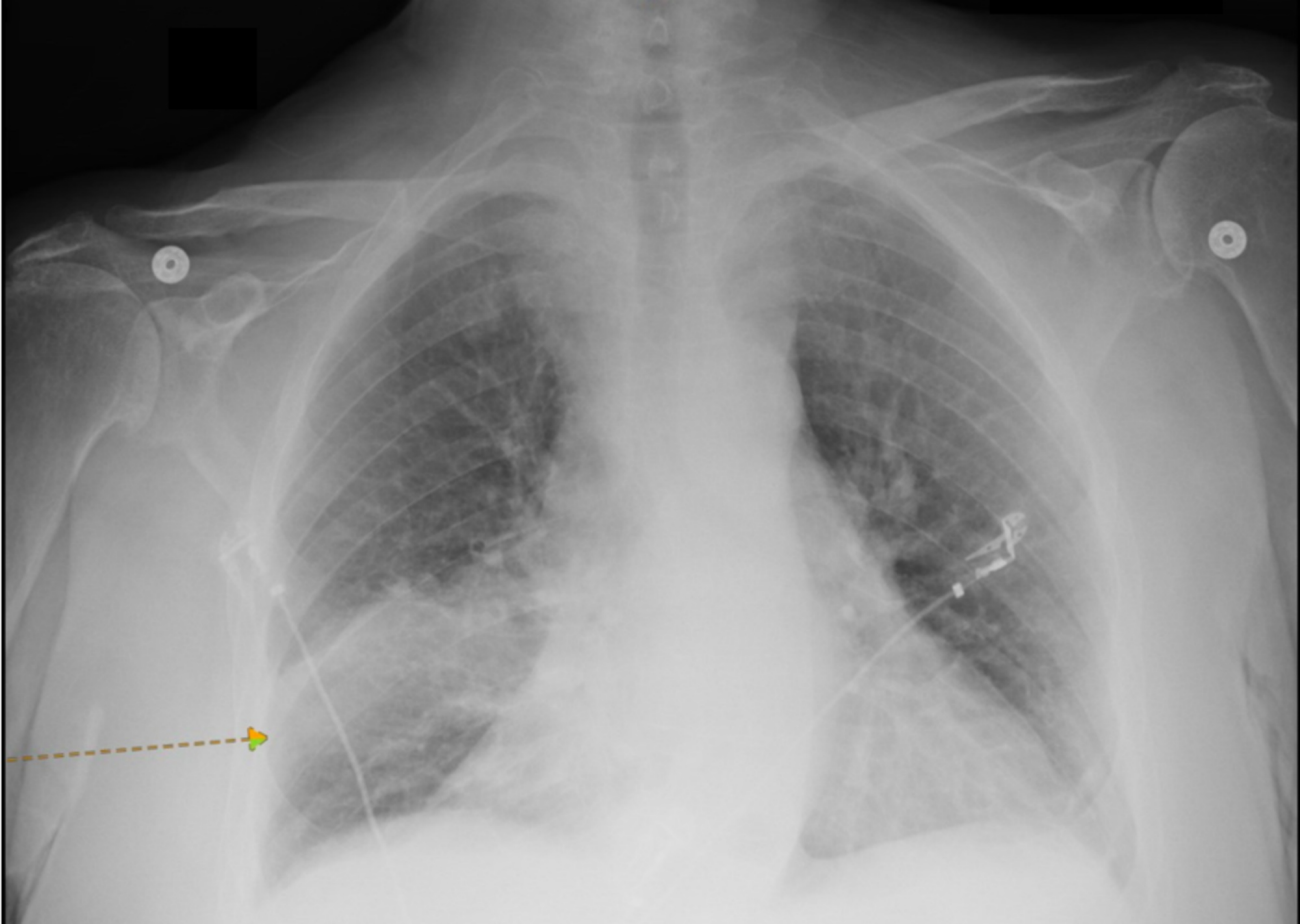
Chest X-ray There is opacity in the right lower lung (arrow).
